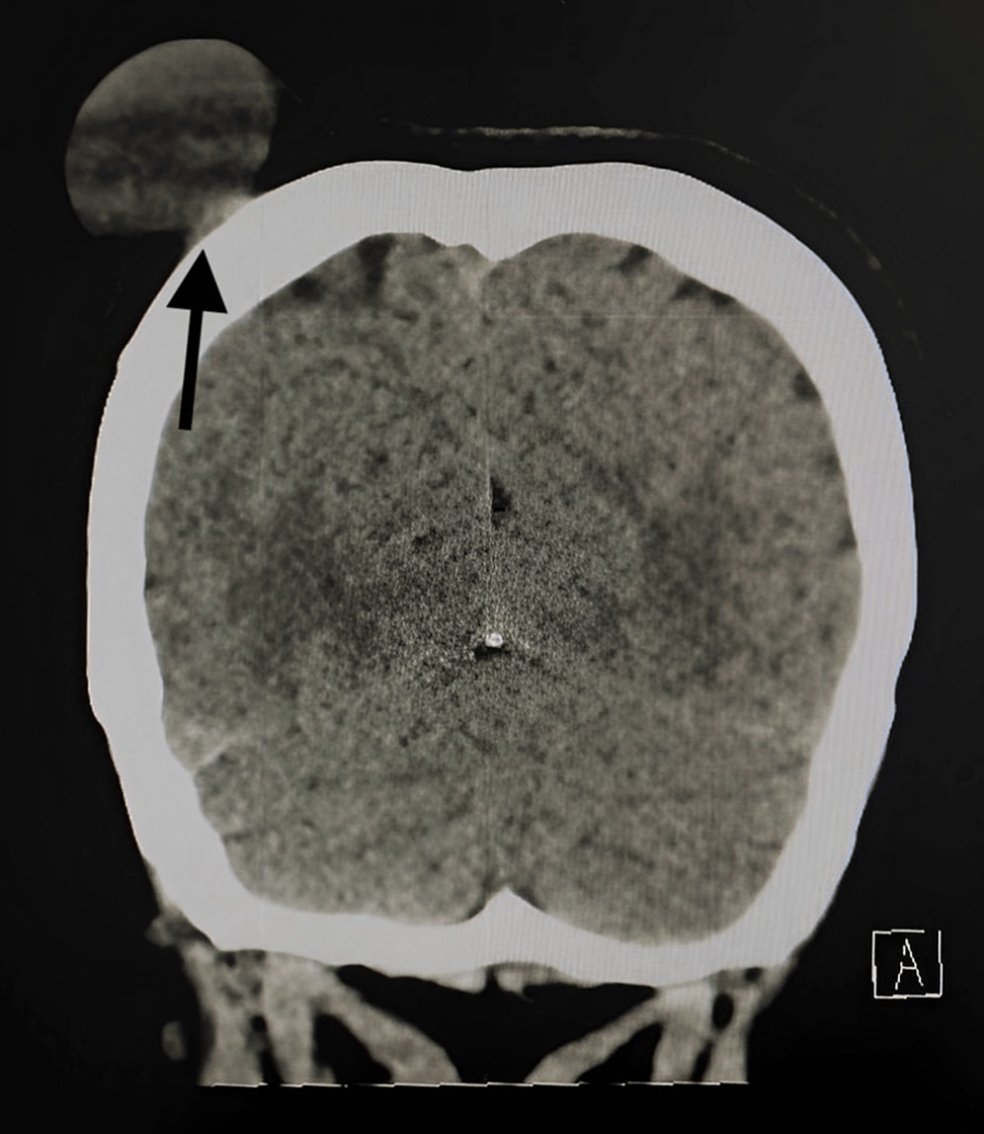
CT scan of the brain without contrast (coronal view) showing significant right scalp swelling with no evidence of bony destruction
